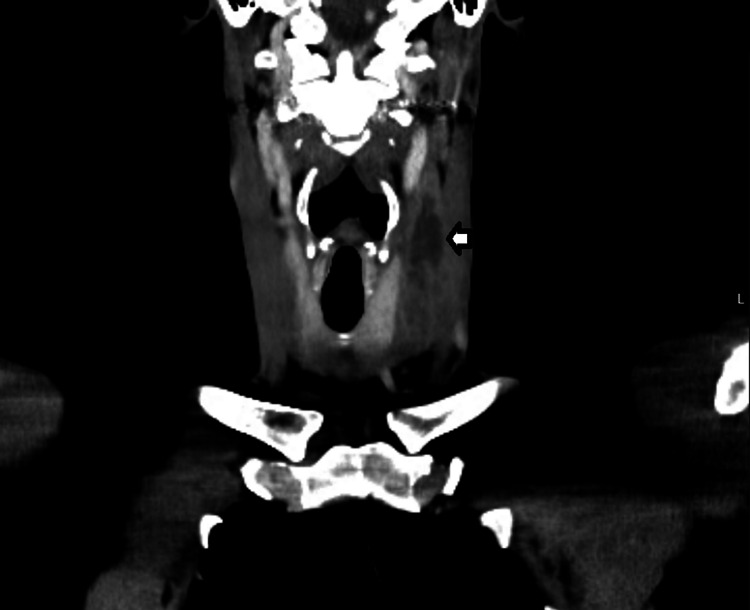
CT neck one week post-anticoagulation The CT neck shows a thrombus within the left internal jugular vein, which appears smaller in size, measuring 2 x 2 x 6.7 cm (white arrow).
